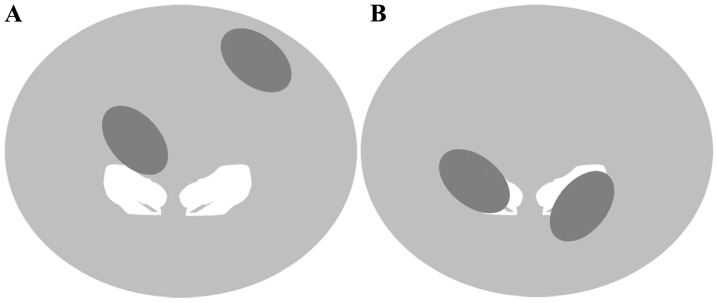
Prostate calcification analysis. The number and position of the prostate calcifications were not evaluated in the present study. (A) When prostate calcification and the FDG uptake area overlapped in less than 40% of the areas, the cases were defined as ‘uptake not coexisting with calcification’. (B) The other cases, i.e. 40% or more of overlapped areas, were defined as ‘uptake coexisting with calcification’.
